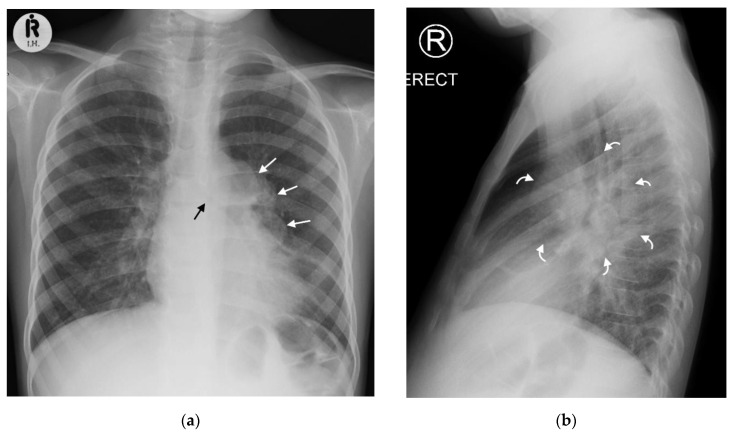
(a,b): Left hilar lymphadenopathy on the PA and lateral chest radiographs. (a) PA erect chest radiograph in this child with later confirmed pulmonary TB demonstrates a multilobulated lymph node mass projecting beyond the cardiac margin on the left (white arrows) consistent with left hilar lymphadenopathy. There is also loss of the left cardiac margin consistent with lingula air-space disease/atelectasis as a consequence of left main bronchus compression (black arrow). (b) Lateral chest radiograph confirms the presence of hilar lymphadenopathy by demonstrating an oval mass consistent with the ‘doughnut sign’ (curved white arrows), representing lymphadenopathy inferiorly and likely the normal vessels (aortic arch and left main pulmonary artery) superiorly.
